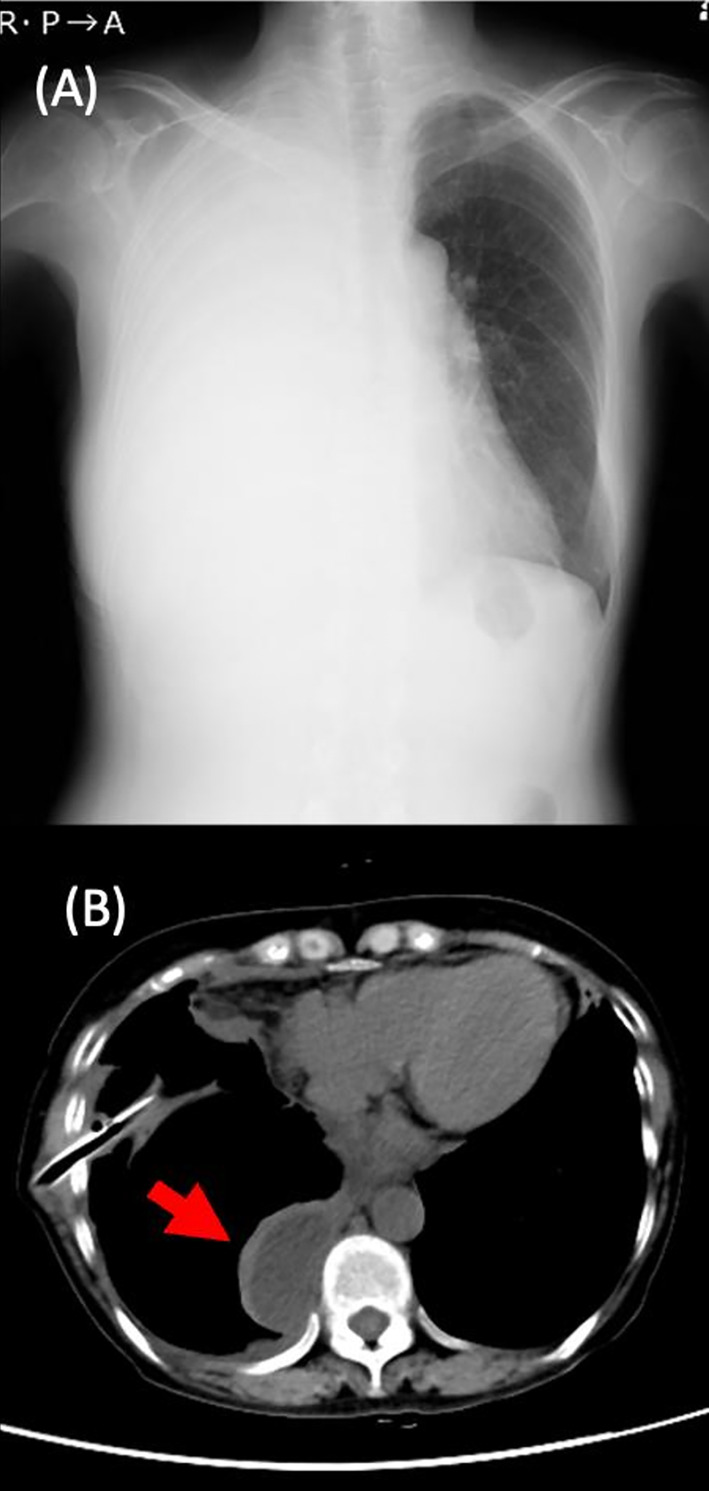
(A) Chest radiograph obtained on the day of admission showing massive right‐sided pleural effusion (23 Dec 20XX‐1). (B) Thoracic computed tomography after chest tube drainage shows an encapsulated pleural effusion (Red arrow) (4 Jan 20XX).
